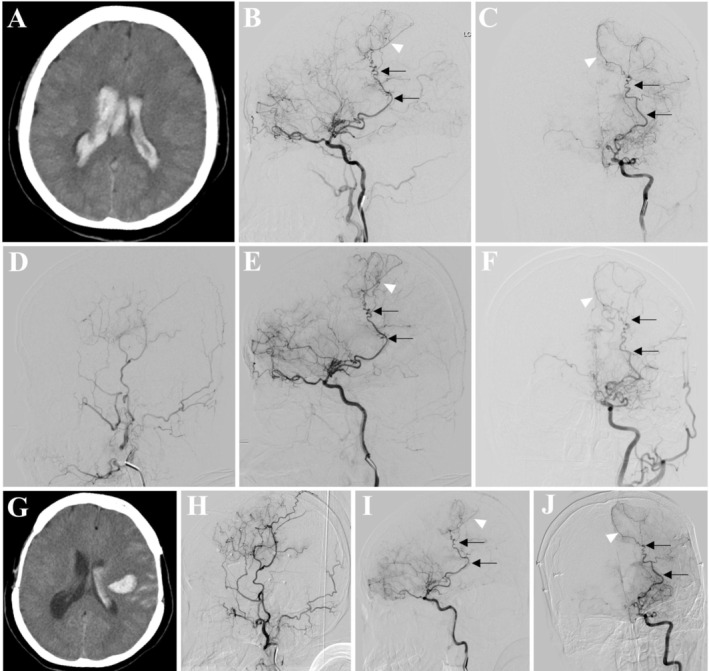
Representative case of rebleeding occurred during follow‐up without PA regression. (A) A 50‐year‐old woman with *RNF213* wild type suffered a sudden onset of intracranial ventricular hemorrhage. (B and C) Lateral (B) and anteroposterior (C) views of baseline left internal carotid angiography show typical findings of choroidal anastomosis, with extreme dilation and extension of the AChoA (black arrows) connecting to the medullary artery (white arrowheads). (D–F) Follow‐up angiography conducted 6 months after surgery showed a poor Matsushima grade (D) and no regression of the dilated and extension of the AChoA (E and F). (G–J) 4.7 years later, the patient suddenly experienced headache and weakness in the right limbs. CT scan revealed de novo hemorrhage located in the left periventricular area of the atrium of the lateral ventricle, consistent with the distribution of the choroidal anastomosis (G). Although subsequent angiography indicated an improvement in the Matsushima grade compared to the previous assessment (H), the dilated and extension of the AChoA had still not regression (I and J).
